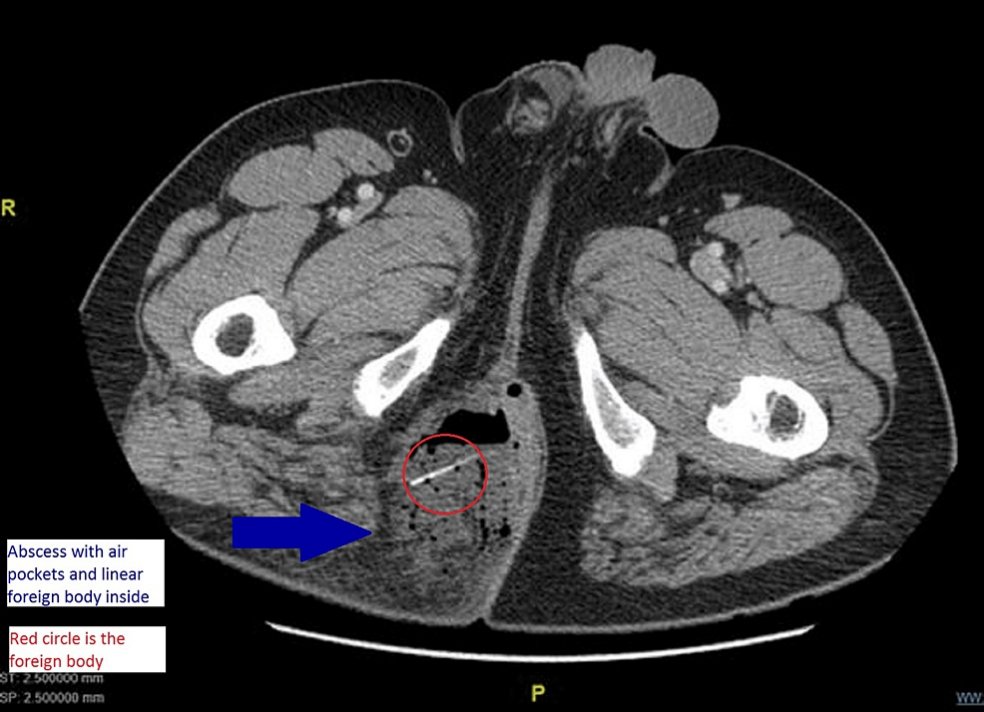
CT scan (axial cut) showing right gluteal abscess with a linear foreign body (red circle)
